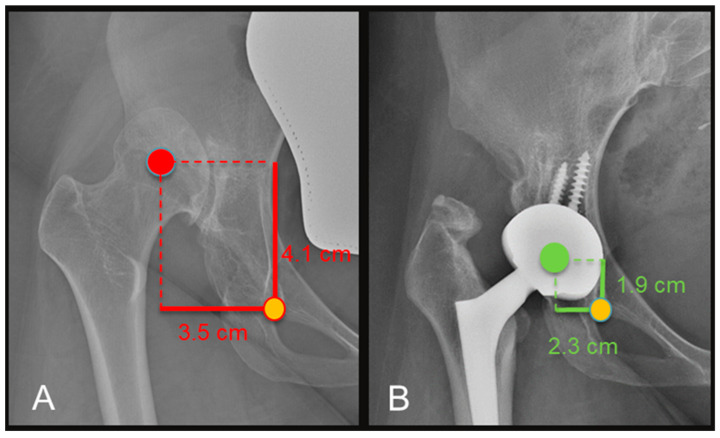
Pre-operative (A) and 18 months (B) X-rays showing medialization and distalization of the COR with a decrease in both horizontal and vertical distance from the teardrop.
